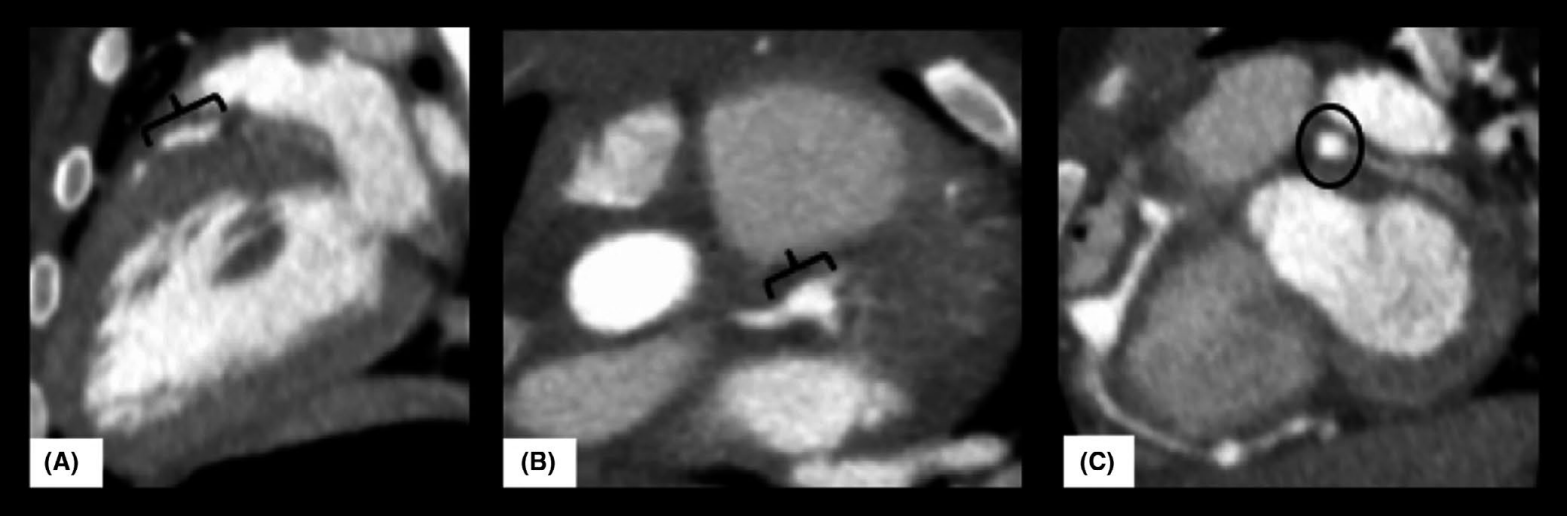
Contrast‐enhanced CT angiographic images of the LMCA and branches. Image A demonstrates a prominent LAD with irregular margins without aneurysmal dilatation of the segment between the bifurcation with the LCX and the first septal branch. Image B in long axis ({) and image C in short axis (○) depict aneurysmal dilatation of the bifurcation of the LAD and LCX, measuring 4.3 × 4.0 × 5.1 mm
